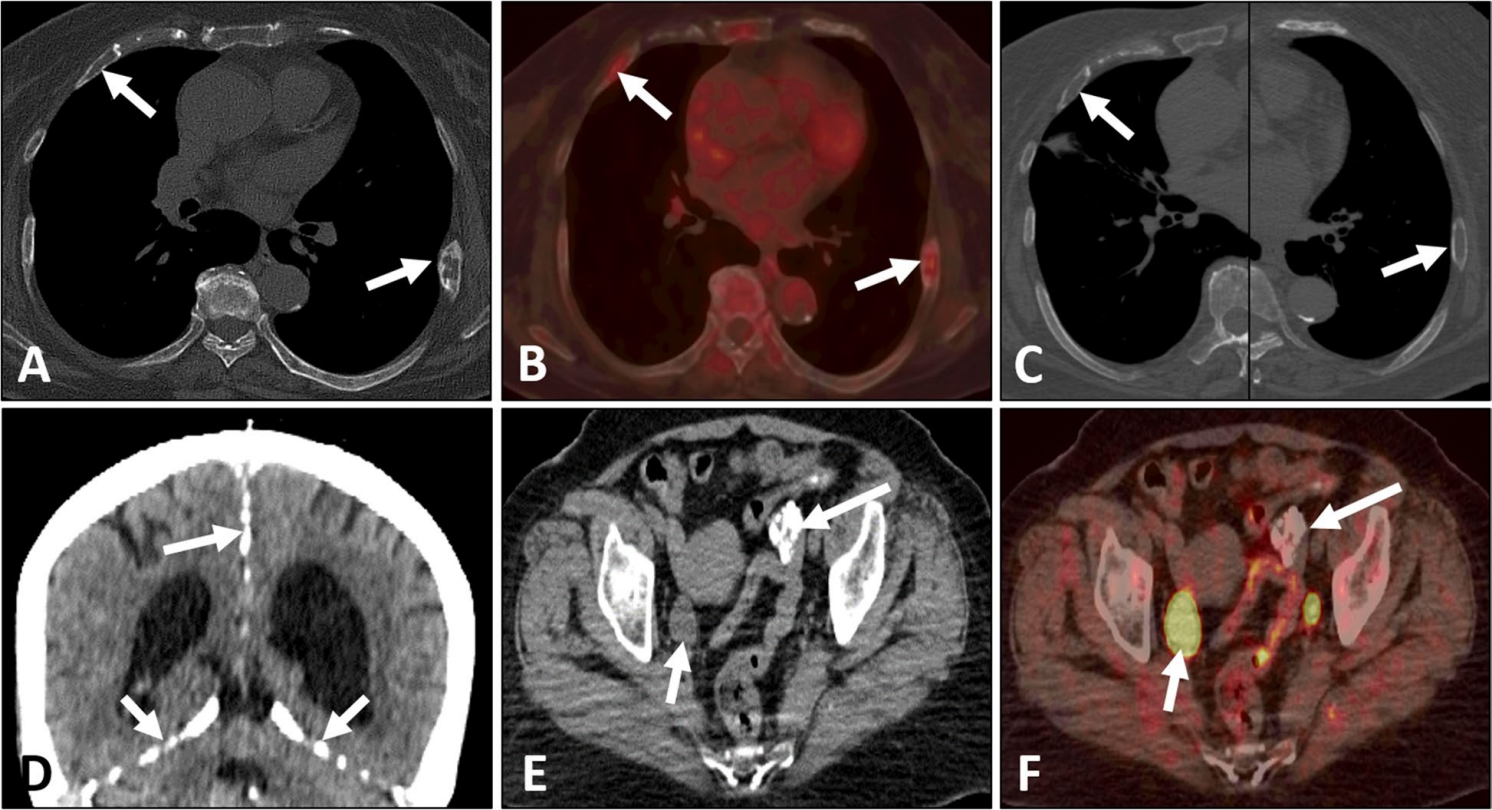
Nevoid basal cell carcinoma syndrome (NBCCS) in a 71-year-old woman with the left invasive breast cancer. The bilateral ribs (arrows) showed bone expansion of cystic lesions and mild thickening of the bone cortex with increased ^18^F-FDG uptake on PET/CT (A: PET; B: PET/CT). Two years ago, the patient's chest CT images showed the bilateral ribs (arrows) had developed cystic changes (C: CT). The falx cerebrum (long arrow) and tentorium cerebellum (short arrows) were accompanied with multiple calcifications (D: CT). The left ovary (long arrow) also developed multiple calcifications without ^18^F-FDG uptake (E: CT; F: PET/CT). The right ureter (short arrow) was dilated (E: CT; F: PET/CT)
